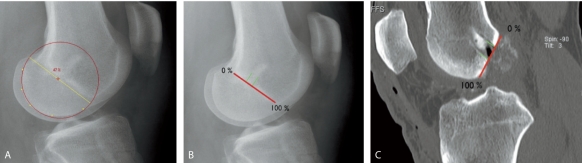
A. Lateral view of the lateral femoral condyle by the method of Amis (Amis et al. 1994). The yellow dots are the user-defined edges of the posterior femoral condyle. A circle is automatically fitted on to the dots and can be rotated, in such a way that the diameter is parallel to Blumensaat's line. B. Lateral view of the lateral femoral condyle by the method of Aglietti (Aglietti et al., 1995). These authors compare the tunnel measurement to a line parallel to the most posterior and anterior part of Blumensaat's line. C. Measurement according to Aglietti on the sagittal femoral CT reconstruction.
